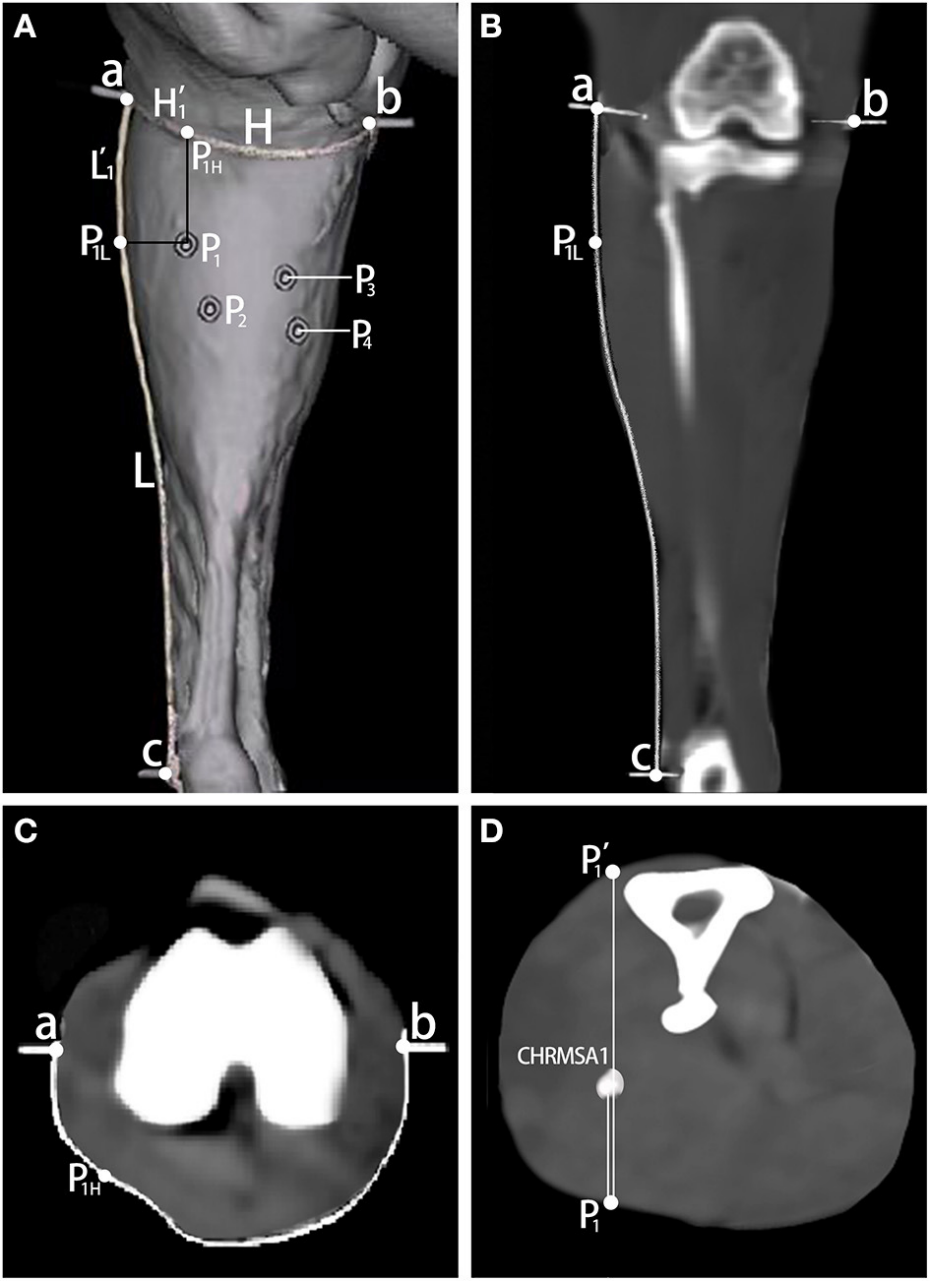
Spiral CT localization of CHRMSA in the lateral head of the gastrocnemius muscle. (A) Projection point of CHRMSA on the body surface and the designed reference lines. (B) Measurement of the lengths of the L line and the L' line on the coronal plane. (C) Measurement of the lengths of the H line and the H' line on the cross-sectional plane. (D) Measurement of the depth of CHRMSA on the cross-sectional plane.
